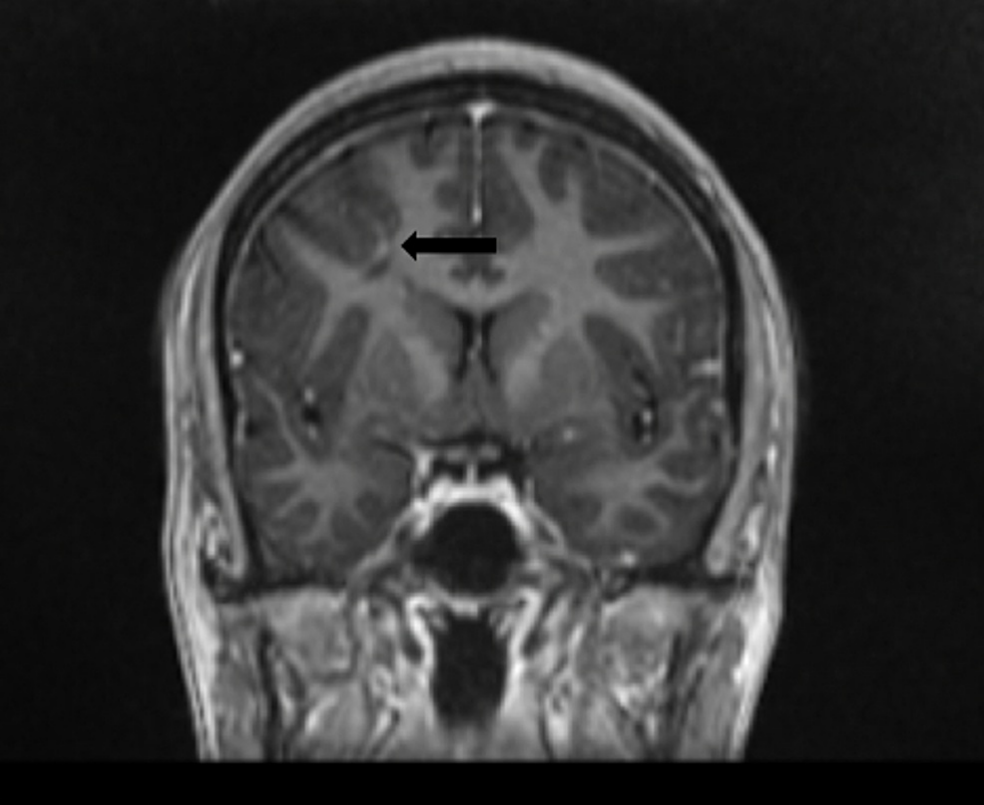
Brain MRI demonstrating a small adjacent developmental venous anomaly (black arrow) adjacent to the right frontal subcortical bright T2/FLAIR hyperintensity.
